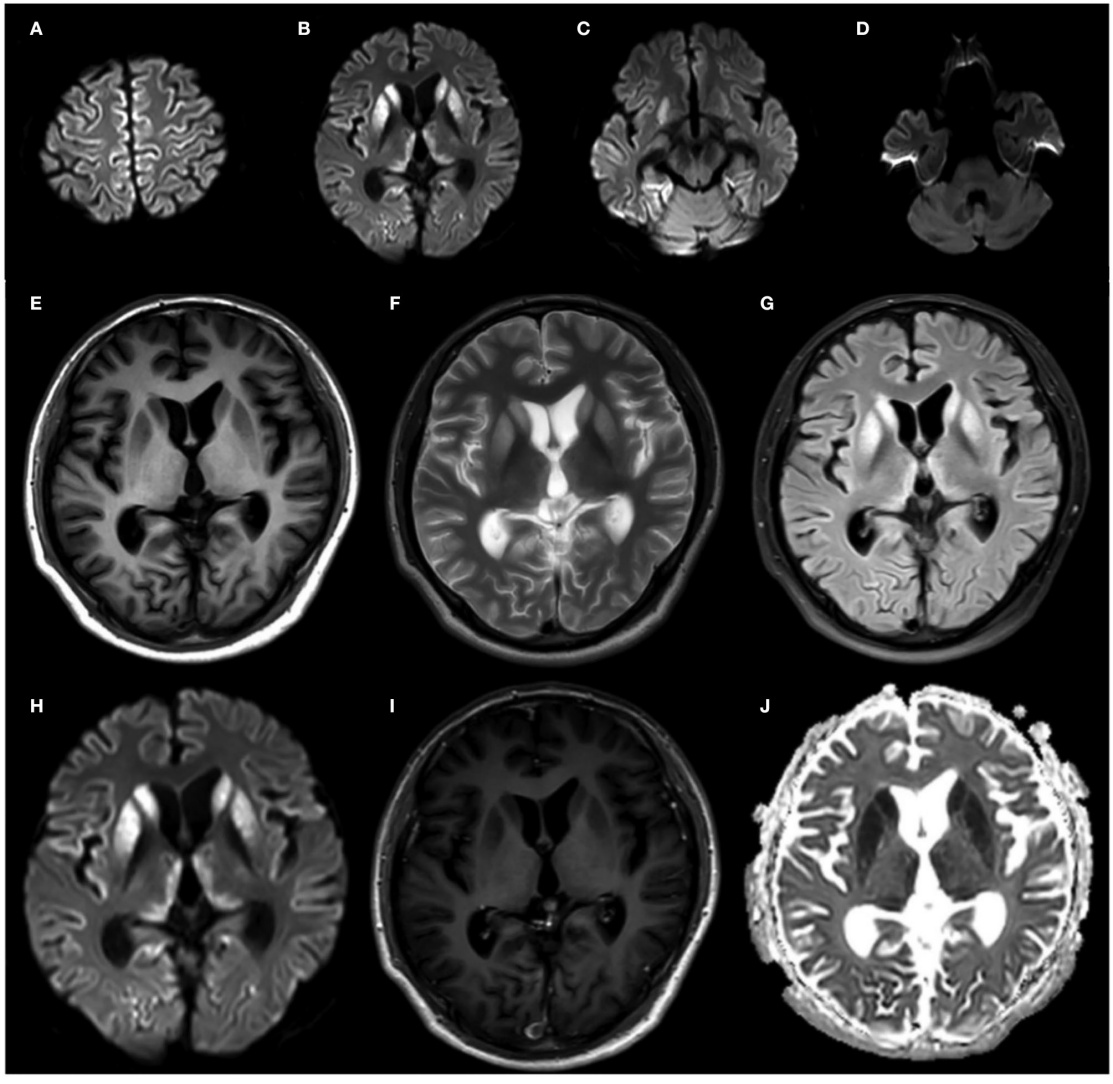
Brain MRI of the proband at 20 months after onset. (A–D) DWI sequences displayed restricted diffusion in the bilateral frontal and parietal cortex. (E–J) Bilateral basal ganglia hyperintensities, with slight bilateral pulvinar and dorsomedial thalamus hyperintensities on FLAIR (I) and DWI (J) sequences.
